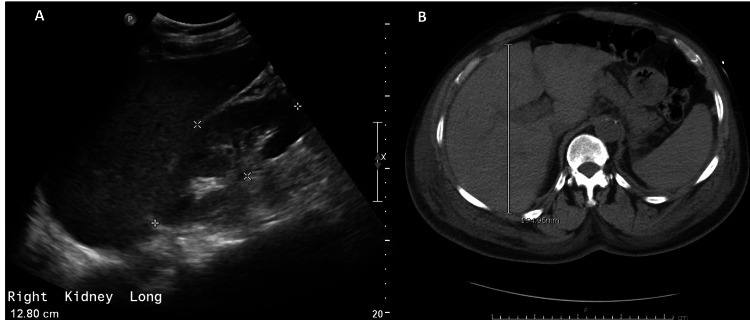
Hepatic assessment: (A) mild hepatic steatosis (abdominal ultrasound); (B) hepatomegaly (liver AP diameter: 19.4 cm) (abdomen CT scan). AP, anteroposterior; CT, computed tomography
